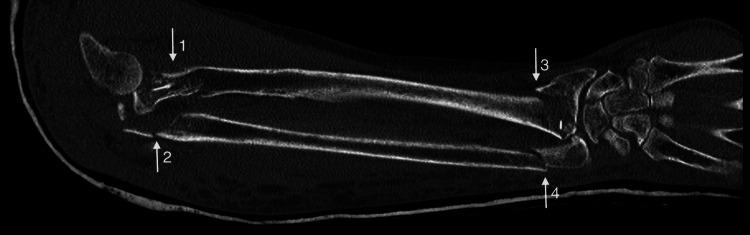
Coronal CT of right forearm Arrow 1: Radial head fracture; Arrow 2: Olecranon fracture; Arrow 3: Distal radius fracture; Arrow 4: Distal ulna fracture
